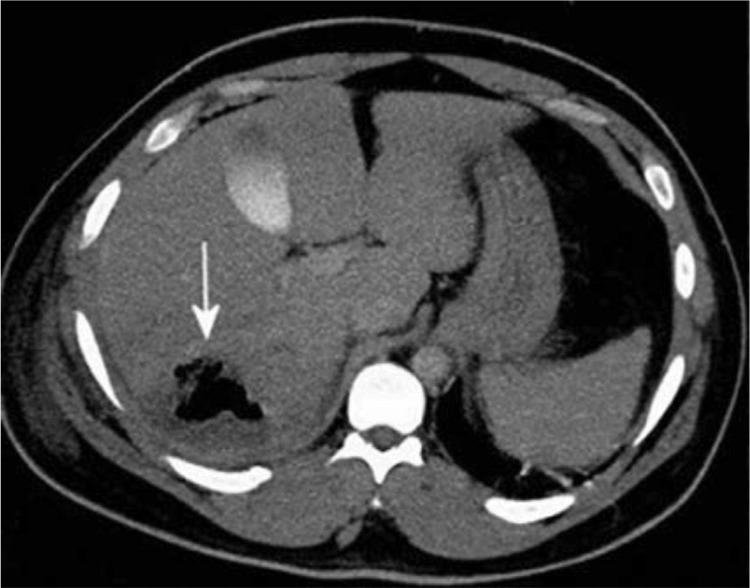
Contrast-enhanced computed tomography axial scan at follow-up showing a huge gas-forming abscess in the right liver lobe (arrow).
